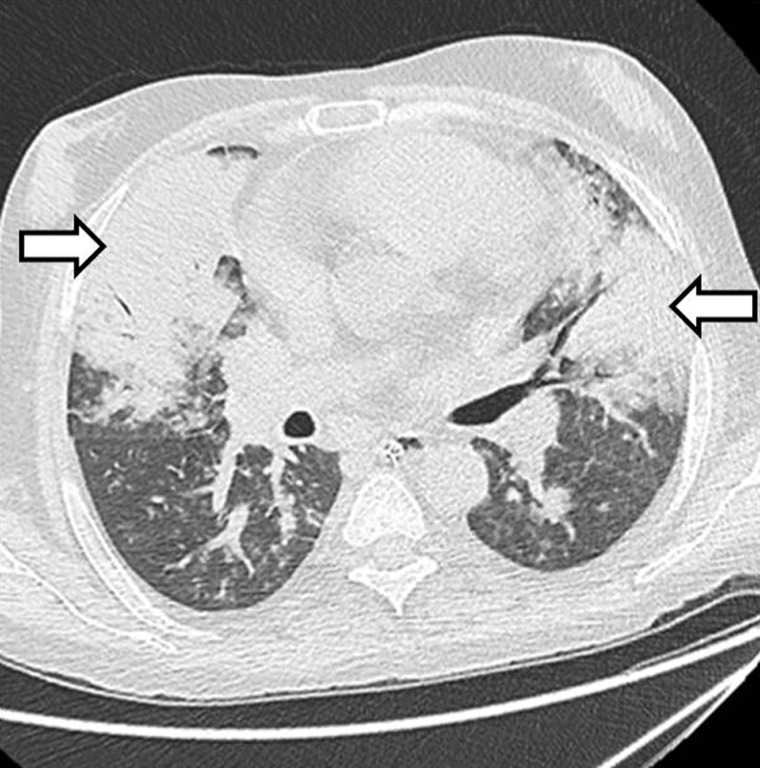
Fungal pneumonia. Axial high resolution CT lung window scan showing *Aspergillus* infection in the form of dense mass-like consolidation (arrows) in bilateral lungs with surrounding areas of ground-glass opacities.
